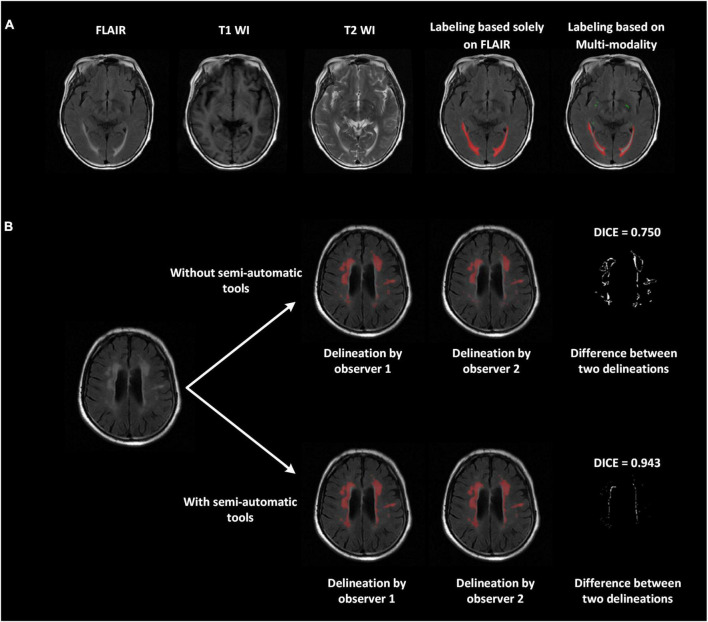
Improved delineation with our labeling strategies. (A) Multi-modality images (T1-weighted, T2-weighted, and FLAIR) applied to differentiate WMH and other intracranial lesions and reduce false positives. The ventricular regions were correctly avoided. The perivascular space, marked in green, was also labeled jointly on all three modalities. (B) With the use of semi-automatic tools, the difference between the two delineations declined, and the Dice improved from 0.75 to 0.943.
